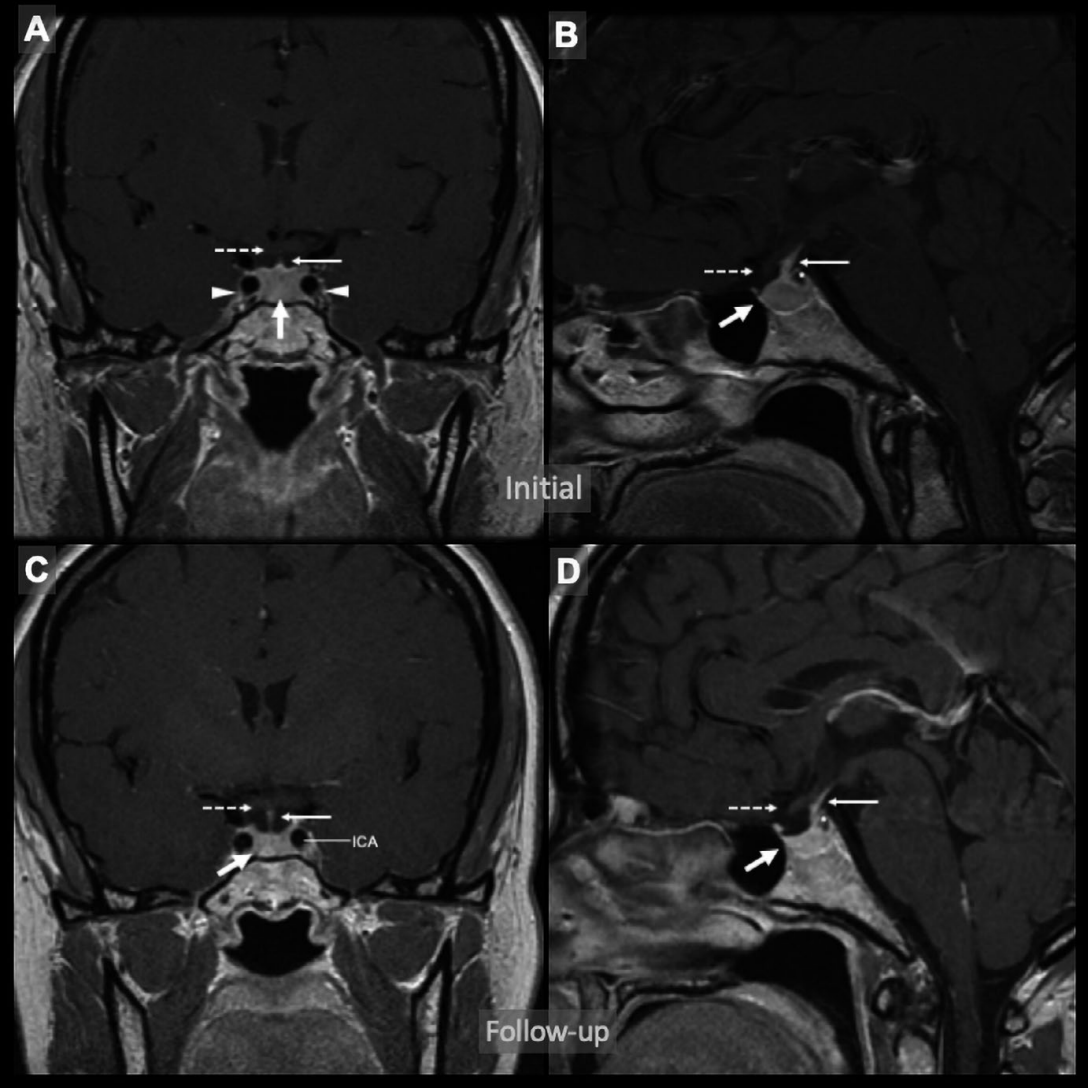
A, Coronal view and (B) sagittal view of the initial contrast‐enhanced magnetic resonance imaging (MRI) of the pituitary gland showing an enlarged and homogeneously enhanced pituitary (thick arrows) measuring 1.1 × 0.8 × 0.8 cm, consistent with pituitary hyperplasia; as well as a cystic focus (asterisk) at the superior portion of the neurohypophysis extending toward the posterior margin of the infundibulum (solid thin arrow) without displacing it. No mass effect on the optic chiasm (dashed thin arrows), no suprasellar extension, or involvement of the cavernous sinuses (arrowheads) identified. C, Coronal view and (D) sagittal view of the follow‐up MRI of the pituitary gland showing a normal‐sized pituitary (thick arrows) along with a stable subcentimeter cystic focus (asterisk) at the superior portion of the neurohypophysis likely representing a pars intermedia or Rathke's cleft cyst. No mass effect on the optic chiasm (dashed thin arrows) or extension into adjacent structures identified. Pituitary infundibulum is indicated with solid thin arrows. ICA, internal carotid artery
